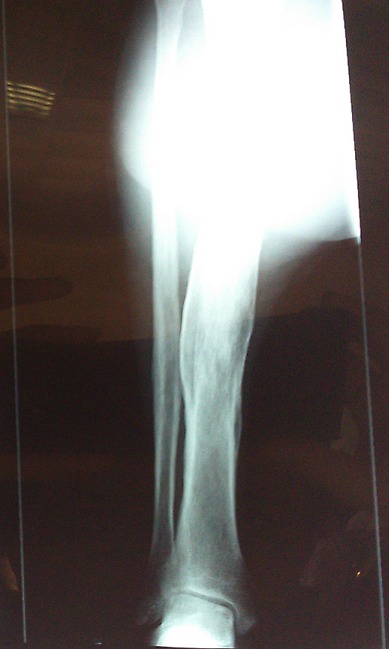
Radiographie standard du membre inférieur: une infiltration des parties molles
